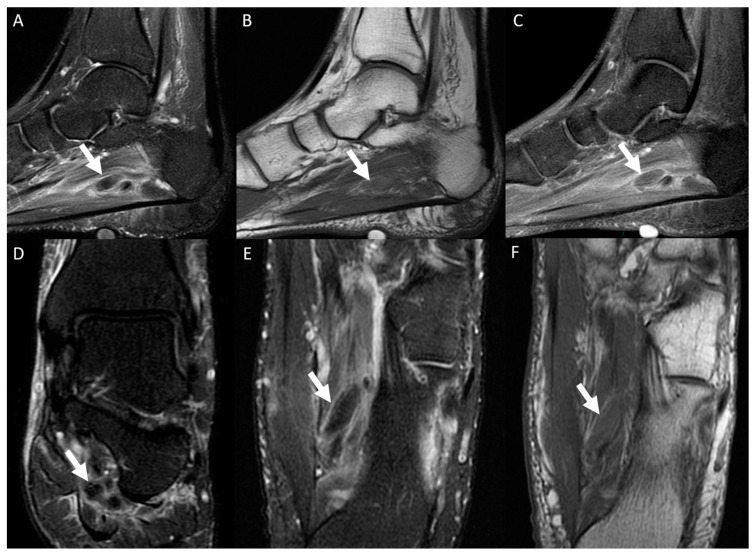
A 45-year-old woman presented with right ankle plantar pain persisting for 2 days, with a clinical suspicion of plantar fasciitis. Ankle MRI images, including a sagittal T2-weighted fat-suppressed scan (A), a T1-weighted scan (B), and a post-gadolinium T1-weighted fat-suppressed scan (C), as well as a coronal T2-weighted fat-suppressed scan (D), a short-axis plane T2-weighted fat-suppressed scan (E), and a T1-weighted scan (F), revealed extensive thrombosis of the lateral plantar vein (arrows). This thrombosis exhibited venous dilation, intraluminal thrombus, and an intravenous filling defect.
